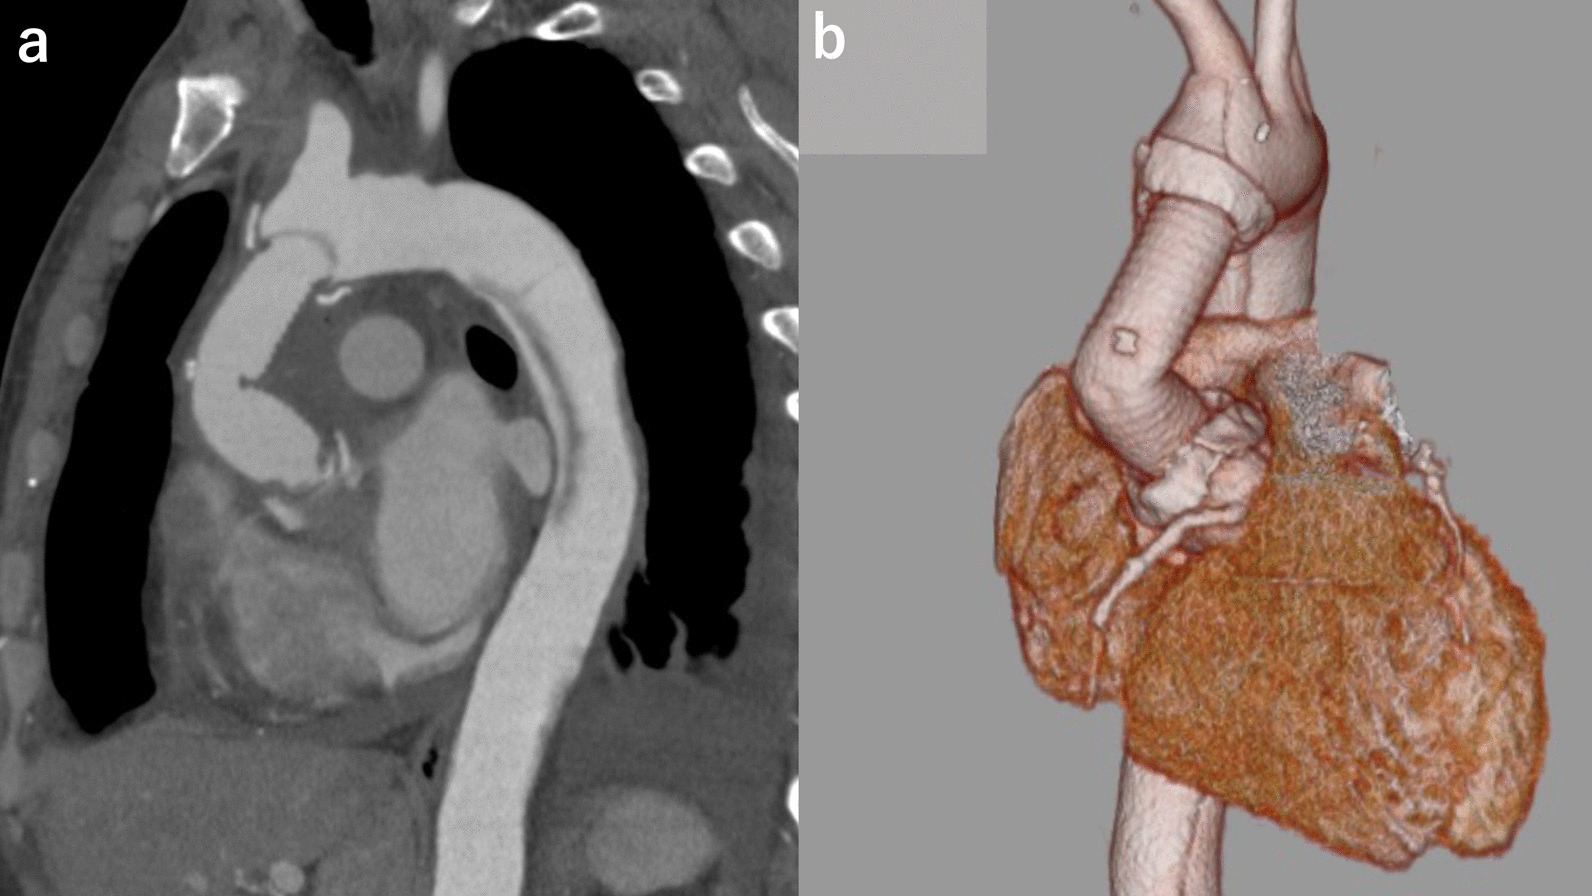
Chest CT scan after initial surgery. a saggital view. b 3D-CT
